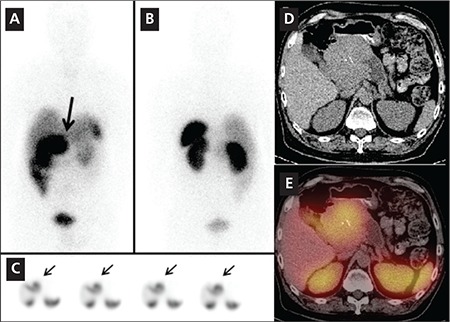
A 50 year-old man had a hyper vascular pancreatic head massdetected on an abdominal CT scan. The patient had an In-111 Pentetreotidescan to evaluate for tumor and to evaluate for distant metastases. Anterior(A) and posterior (B) whole body planar images from an In-111Pentetreotide scan showed a focus of intense abnormal tracer uptake in themid upper abdomen seen on anterior whole body image (arrow, A). Serialaxial SPECT slices (C) at the level of the abnormal tracer uptake (arrows)could not accurately localize the activity. The location could be falselyinterpreted as within the liver, small or large bowel or could be a metastaticupper abdominal mass. Axial CT scan at the level of the left adrenal glandshows a large, centrally calcified pancreatic head mass (D). Fused axial CTimage of SPECT/CT (E) at the same level shows increased tracer uptake inthe pancreatic head mass
